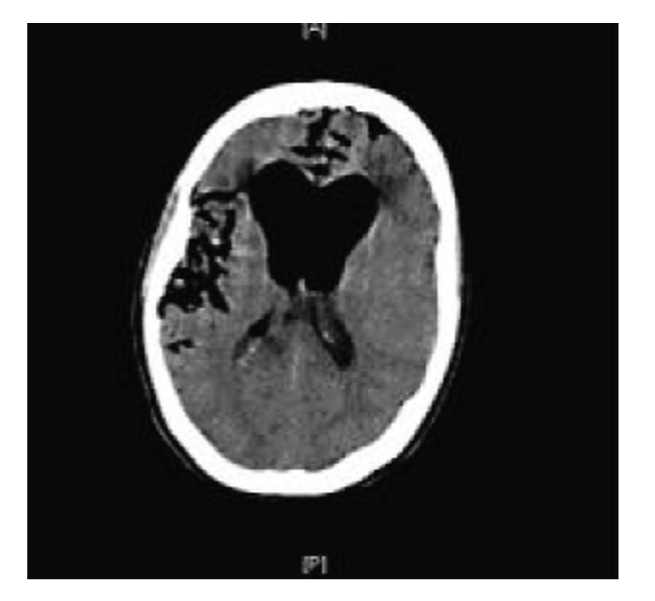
Noncontrast CT head scan showing extensive intraventricular and subarachnoid air.
